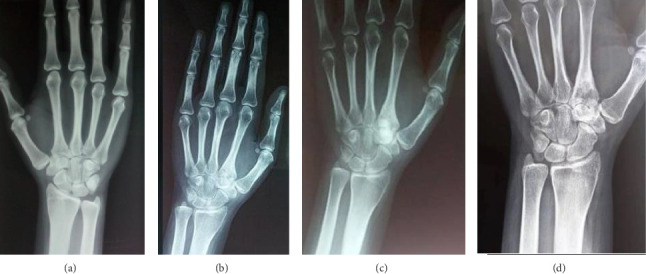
The Evolution of wrist radiography on the time. (a) Normal initial radiography, (b) joint space narrowing of the trapezoid and the second metacarpal bones, (c) well limited sclerotic lesions in the trapezoid and the base of the second metacarpal bones, (d) altering bone at the trapezoid and the second metacarpal bone.
